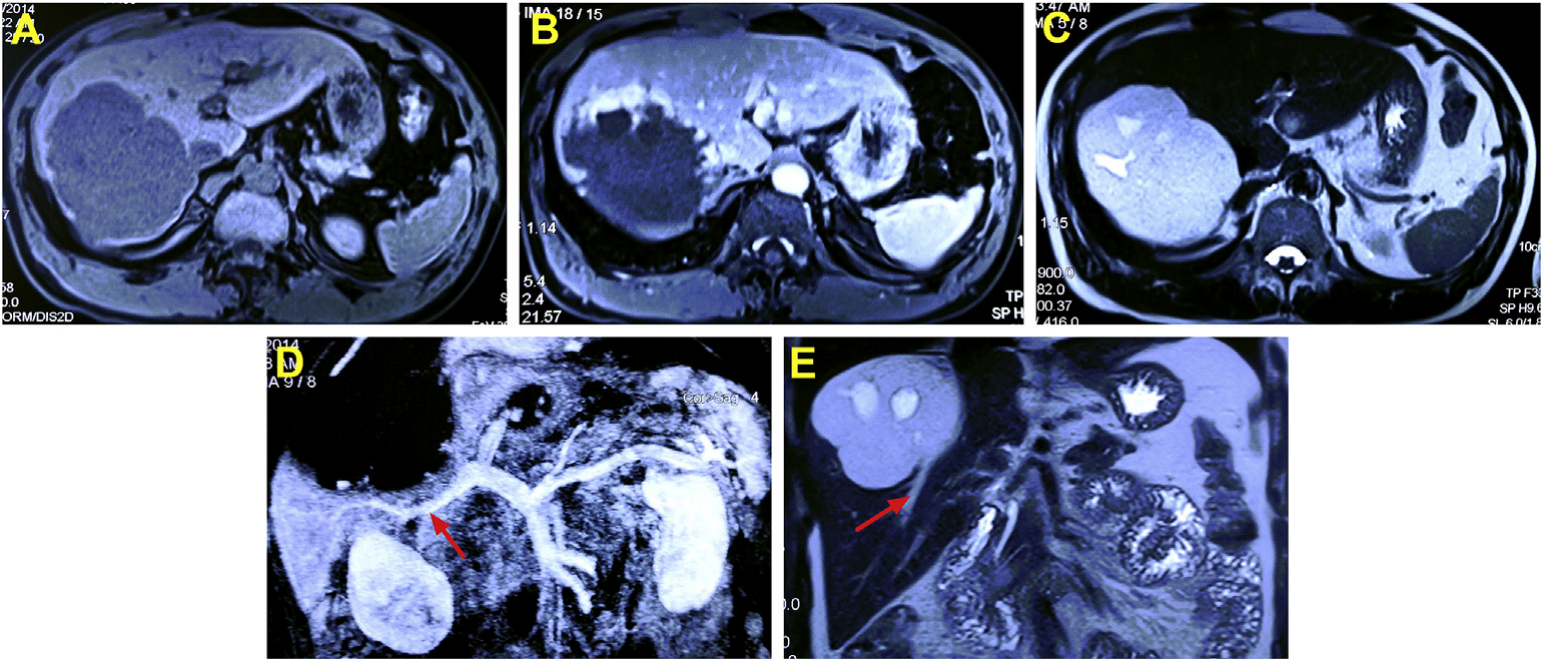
A 54-year-old man had a large hepatic hemangiomas in the right lobe (12.0 cm) as revealed by abdominal MRI scans (A–C). The ablation start from the main blood vessel in the areas of lesions (D, E, red arrow). (For interpretation of the references to color in this figure legend, the reader is referred to the Web version of this article.)
